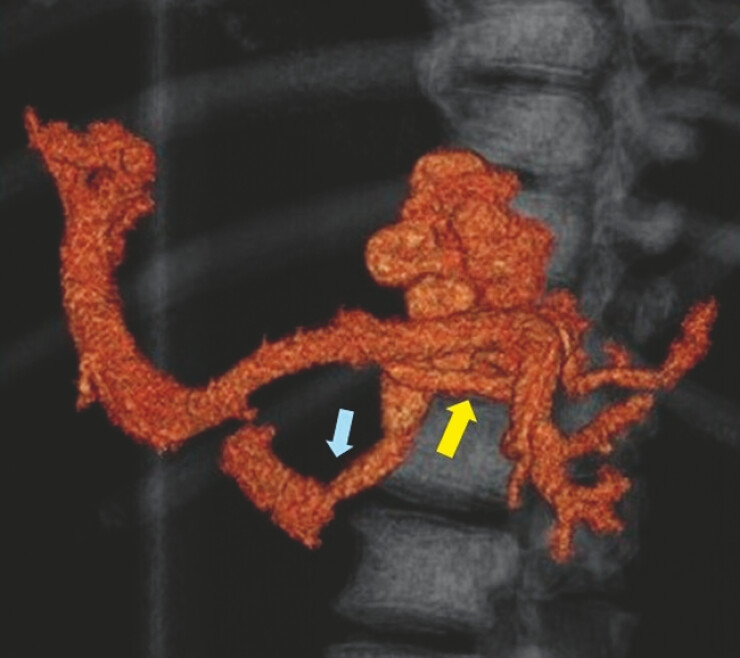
3D-CT revealed the hemodynamics of varices fed from the posterior gastric vein (yellow arrow) through the gastric varices to the gastrorenal shunt (blue arrow). 3D-CT, three-dimensional contrast-enhanced computed tomography.
